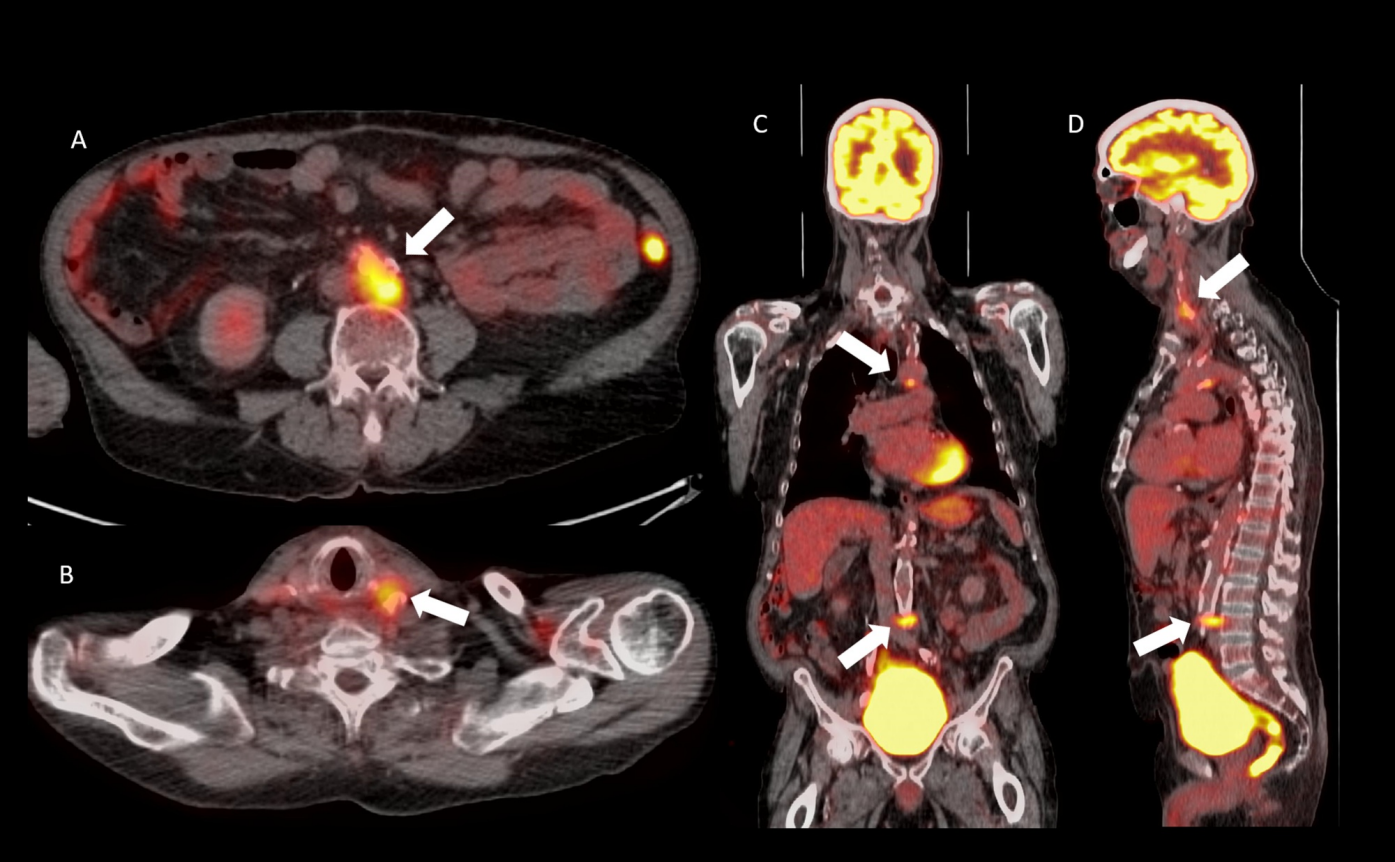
Axial positron emission tomography/computed tomography fusion image (A), axial computed tomography image (B), coronal (C), and sagittal (D) positron emission tomography/computed tomography fusion images demonstrating fluorodeoxyglucose avid atherosclerotic plaques in the regions of the aortic bifurcation, aortic arch, and left carotid (white arrows).
